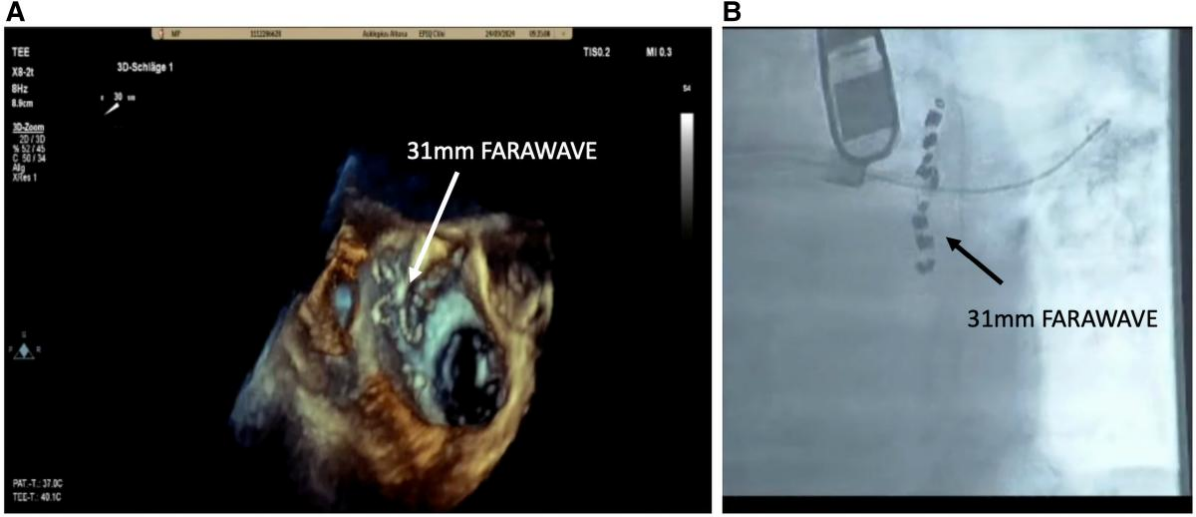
Pulsed field ablation–based pulmonary vein isolation utilizing the FARAPULSE system. 3D transoesophageal echocardiography image (*A*) and fluoroscopy image (*B*) showing the Farawave catheter in the flower position at the ostium of the left inferior pulmonary vein.
